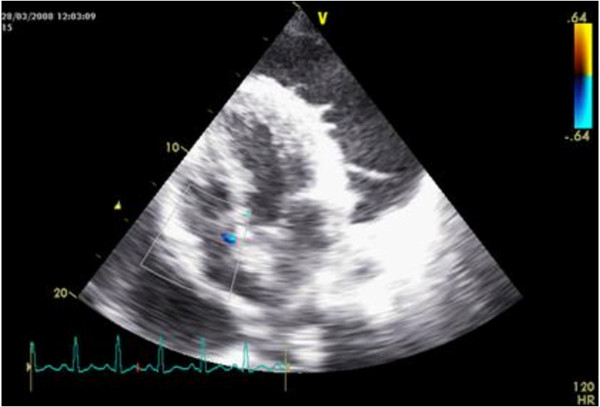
An apical four chamber view of the heart showing frond-like projections from the surface of the left ventricle to pericardial fluid in a patient with tuberculous pericarditis.
